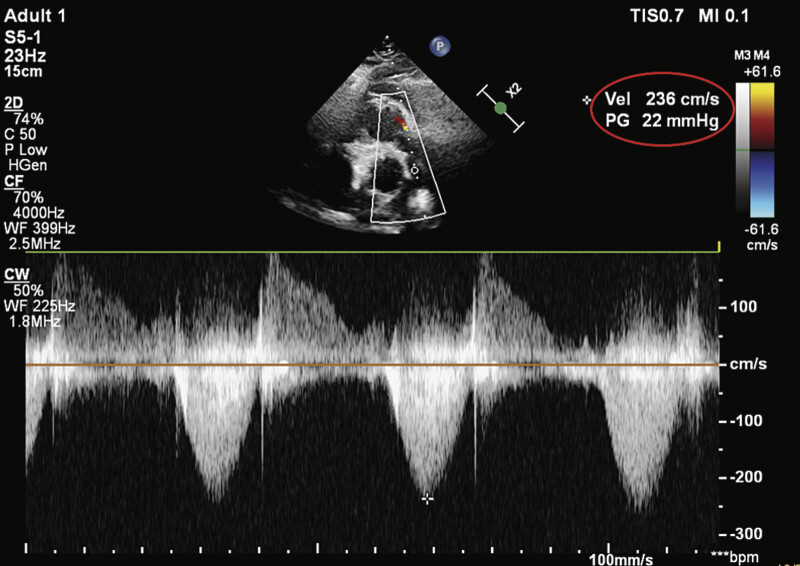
Postoperative echocardiography 30-day postoperative reveals the gradient across the pulmonary homograft.
